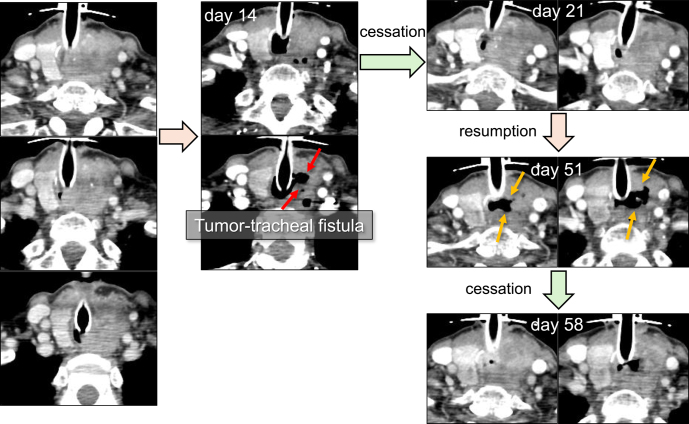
Tumor-tracheal fistula caused by LEN treatment in a patient with ATC (case 2). Tumor-tracheal fistula was first observed 14 days after LEN initiation on CT scan. Since tumor-tracheal fistula was restored by tumor regrowth after 7 days of LEN treatment cessation, LEN treatment was resumed. After resumption of LEN treatment, tumor-tracheal fistula relapsed, and the patient required re-cessation of LEN treatment.
